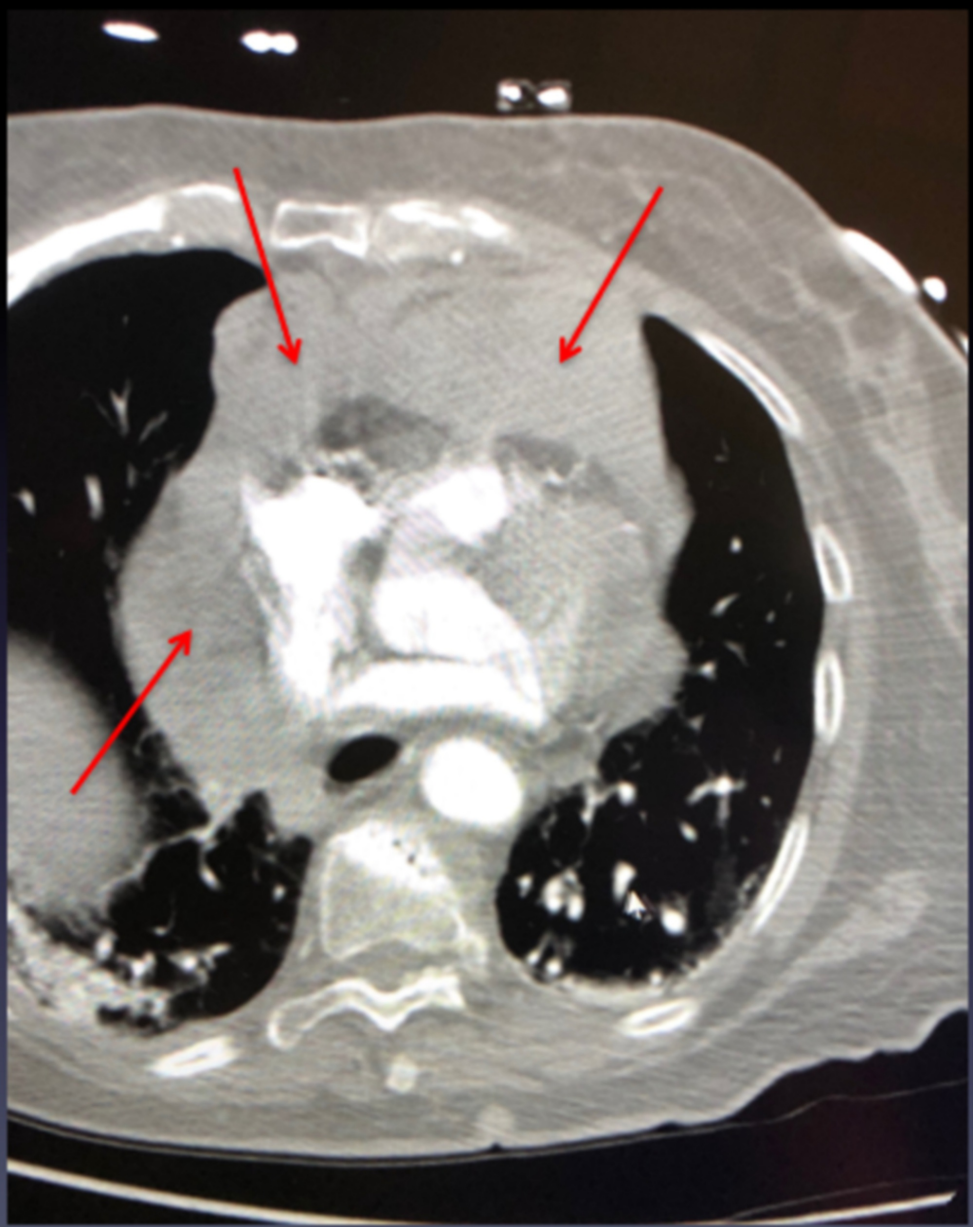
Axial contrast chest CT showing mediastinal hematomas following subclavian artery perforation.
